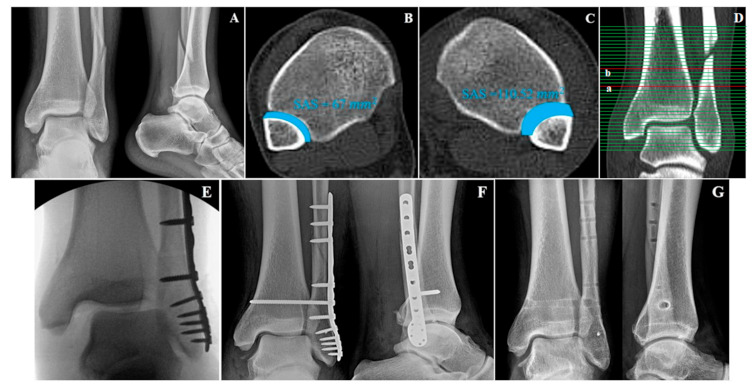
Thirty-seven-year-old male with left ankle fracture. Preoperative anterior-posterior (AP) and lateral radiographs (A). Bilateral axial computed tomography (CT) scan of intact (B) and injured ankle (C) with surface area of syndesmosis (SAS) measured. The ratio of his SAS (injured/intact) was 1.65. Corresponding coronal section (D) at level of 1.0 cm (a) and 1.5 cm (b) above the tibial plafond. Intraoperative fluoroscopic image (E) during stress test demonstrates possible syndesmotic injury. Immediate postoperative AP and lateral radiographs with syndesmosis transfixation included. (F) Last follow up of AP and lateral radiographs (G) with implanted devices removed without diastasis recurrence 11 months later.
